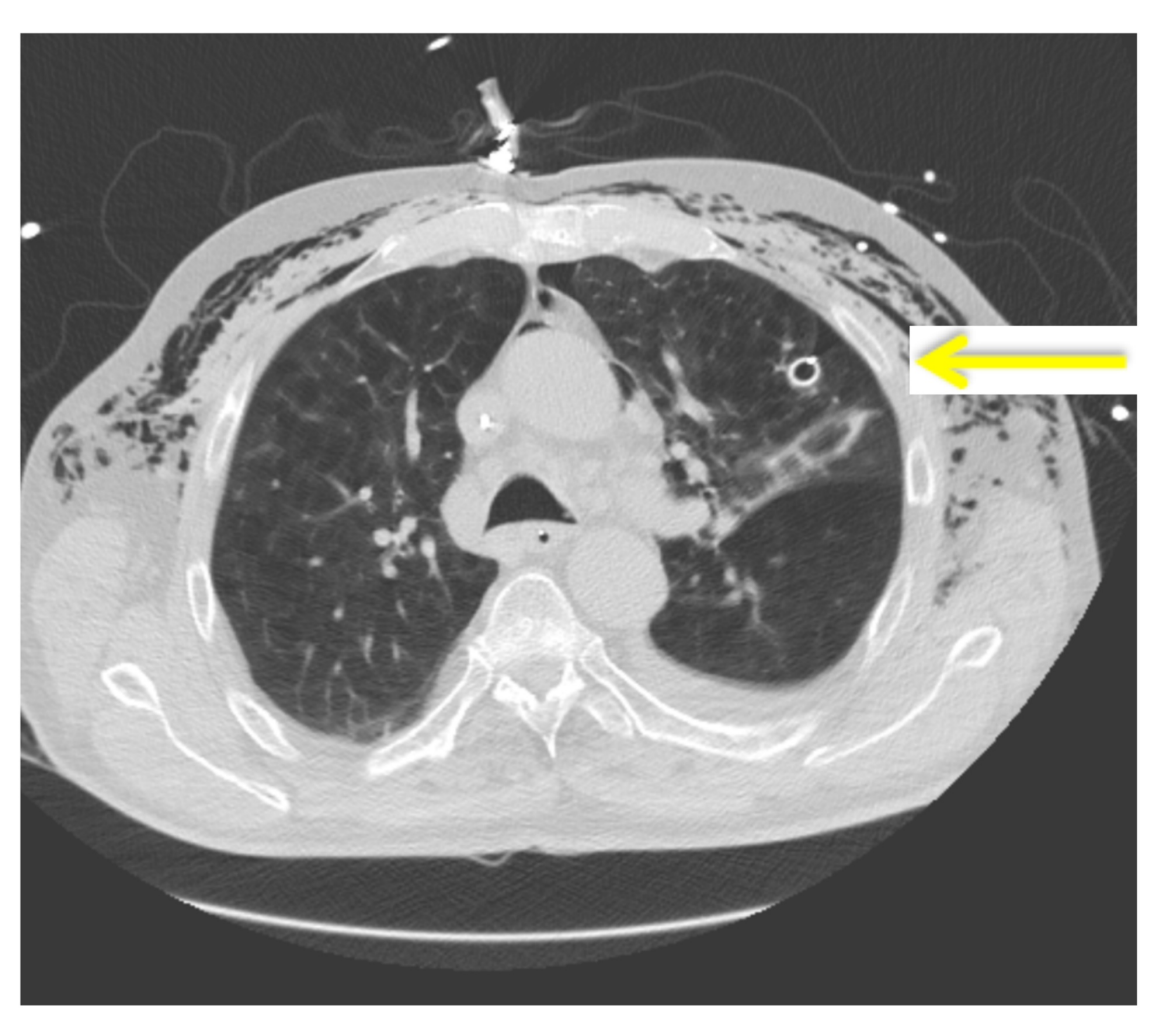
CT scan of the thorax showing the intrapleural location of the thorax drain. The yellow arrow indicated the intrapleural location of the thorax drain
